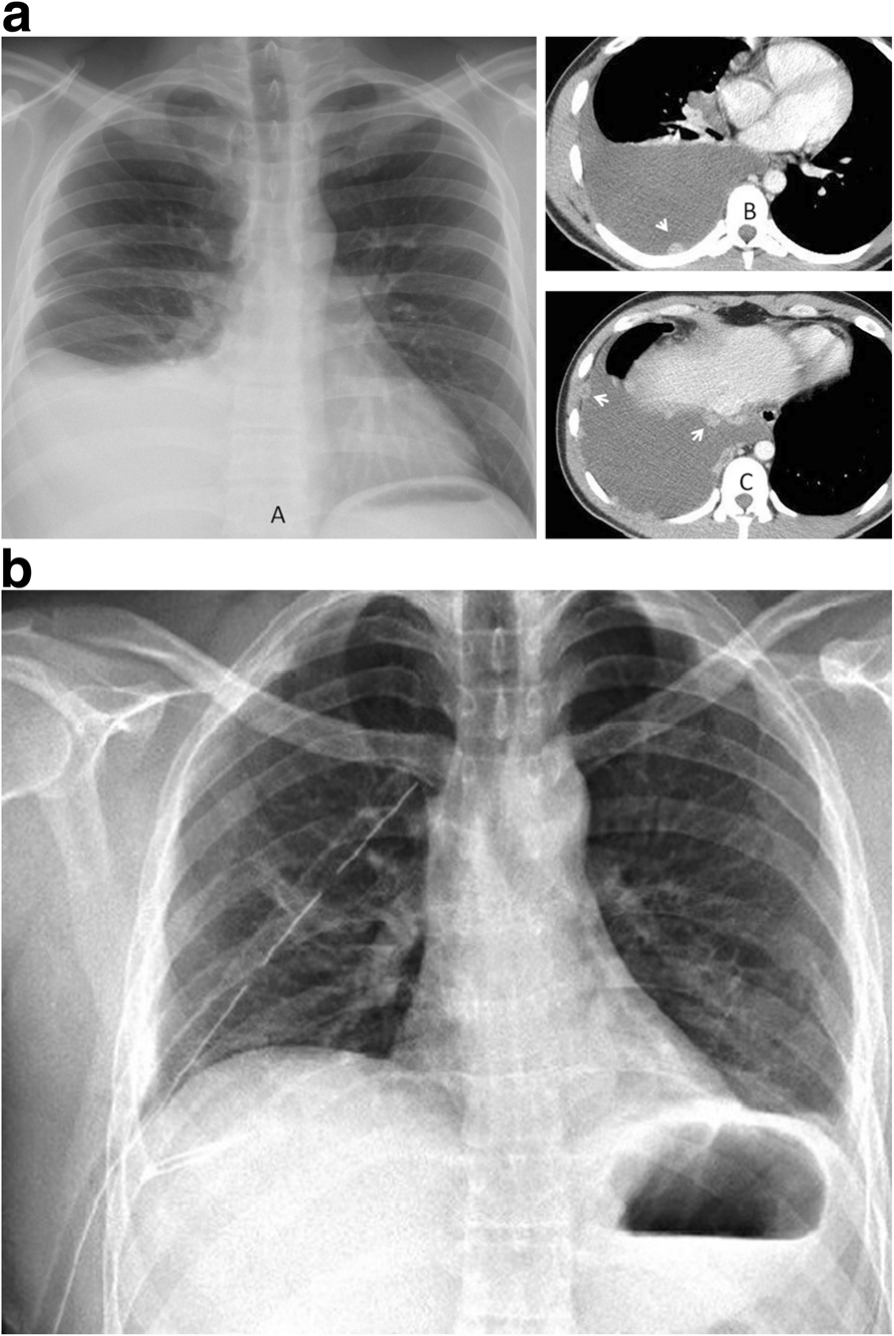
a Radiograph & computed tomography (CT). b Post chest tube radiograph
